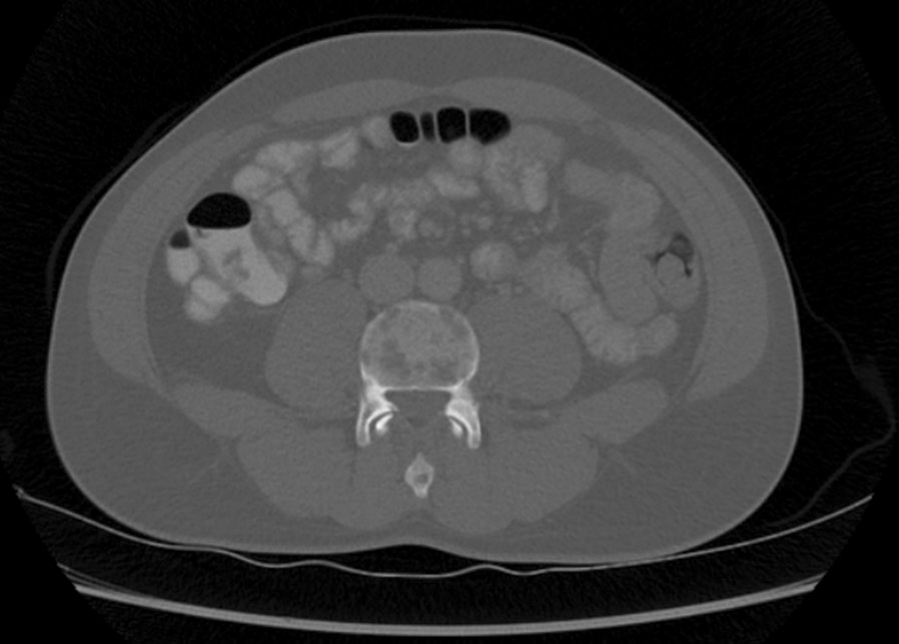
CT scan showing multiple osteolytic lesions of lumbar vertebrae and pelvis
